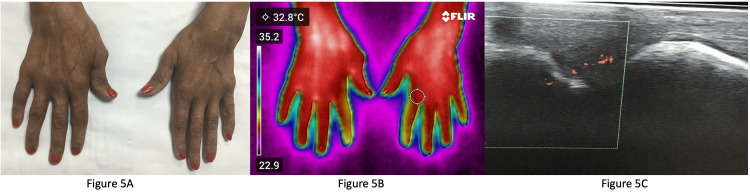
Image of a patient in clinical remission 5A: Clinical image of a patient who was in clinical remission with no active joints clinically; 5B: Infrared thermography of the same patient showing increased temperature at the bilateral wrist and the right first metacarpophalangeal (MCP) joints; 5C: Synovitis with power doppler uptake in the right first MCP joint.
